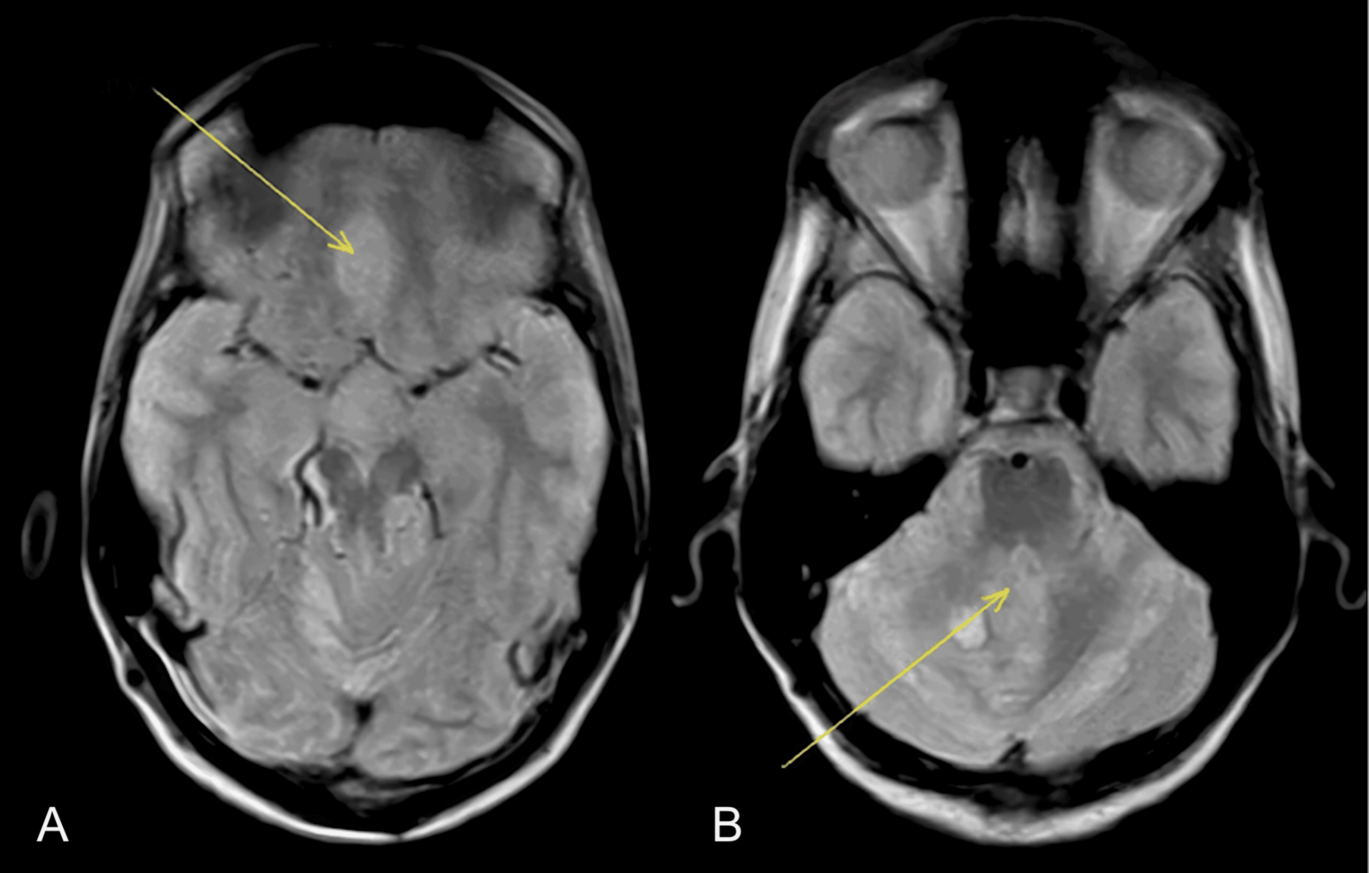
Brain MRI performed one year after surgery. Axial brain MRI demonstrating recurrent posterior fossa tumor and suprasellar metastatic involvement. A: Heterogeneously enhancing mass centered in the posterior fossa with mass effect on the cerebellum and brainstem (yellow arrow). B: Suprasellar enhancing lesion with involvement of the optic chiasm (yellow arrow), consistent with intracranial metastatic dissemination.
